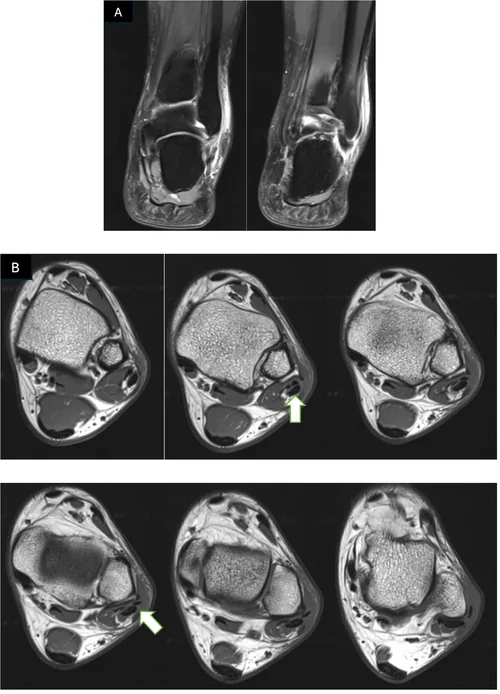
Preoperative magnetic resonance imaging of the left ankle showing (A) coronal and (B) axial views. The images show peroneal tendon tenosynovitis without evident tendon dislocation or subluxation, highlighting the limitation of static imaging in detecting dynamic peroneal instability.
